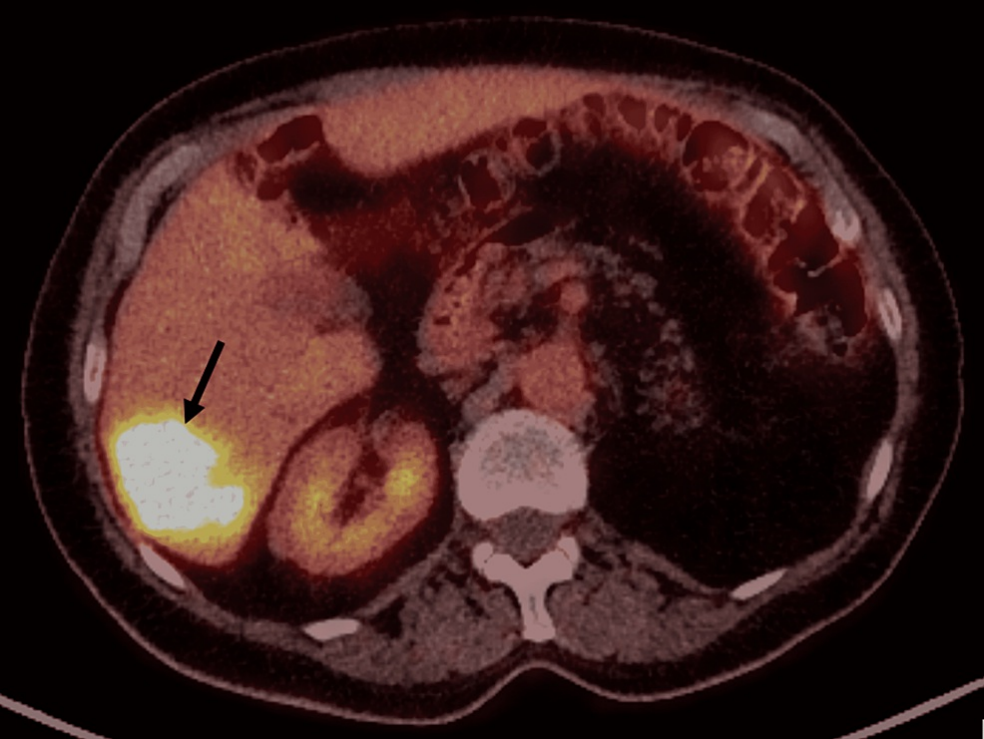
Outpatient PET-CT (pre-SIRT). PET-CT performed pre-SIRT showing solid FDG avid tumour (black arrow) with an SUVmax of 18.6. PET-CT: positron emission tomography-computed tomography; SIRT: selective internal radiation therapy; FDG: fluorine-18-labelled fluorodeoxyglucose; SUVmax: maximum standardized uptake value
